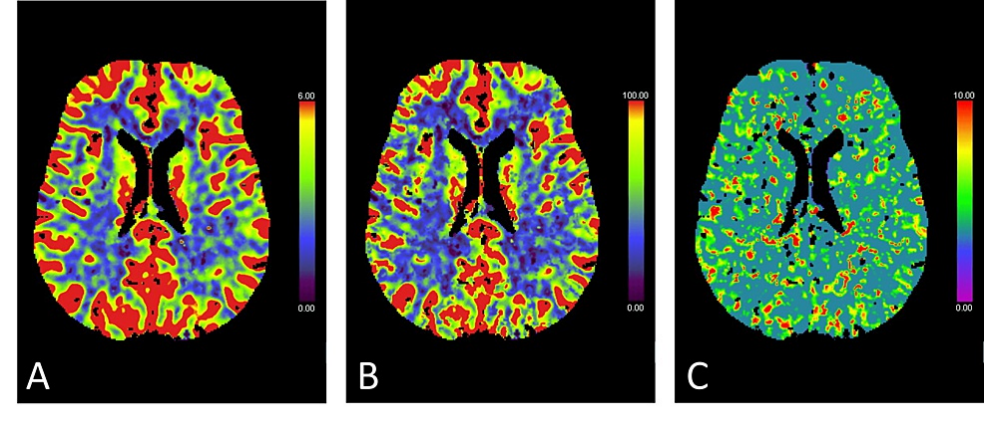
CT perfusion images (A: cerebral blood flow, B: cerebral blood volume, C: mean transit time) revealing no brain ischemia.
